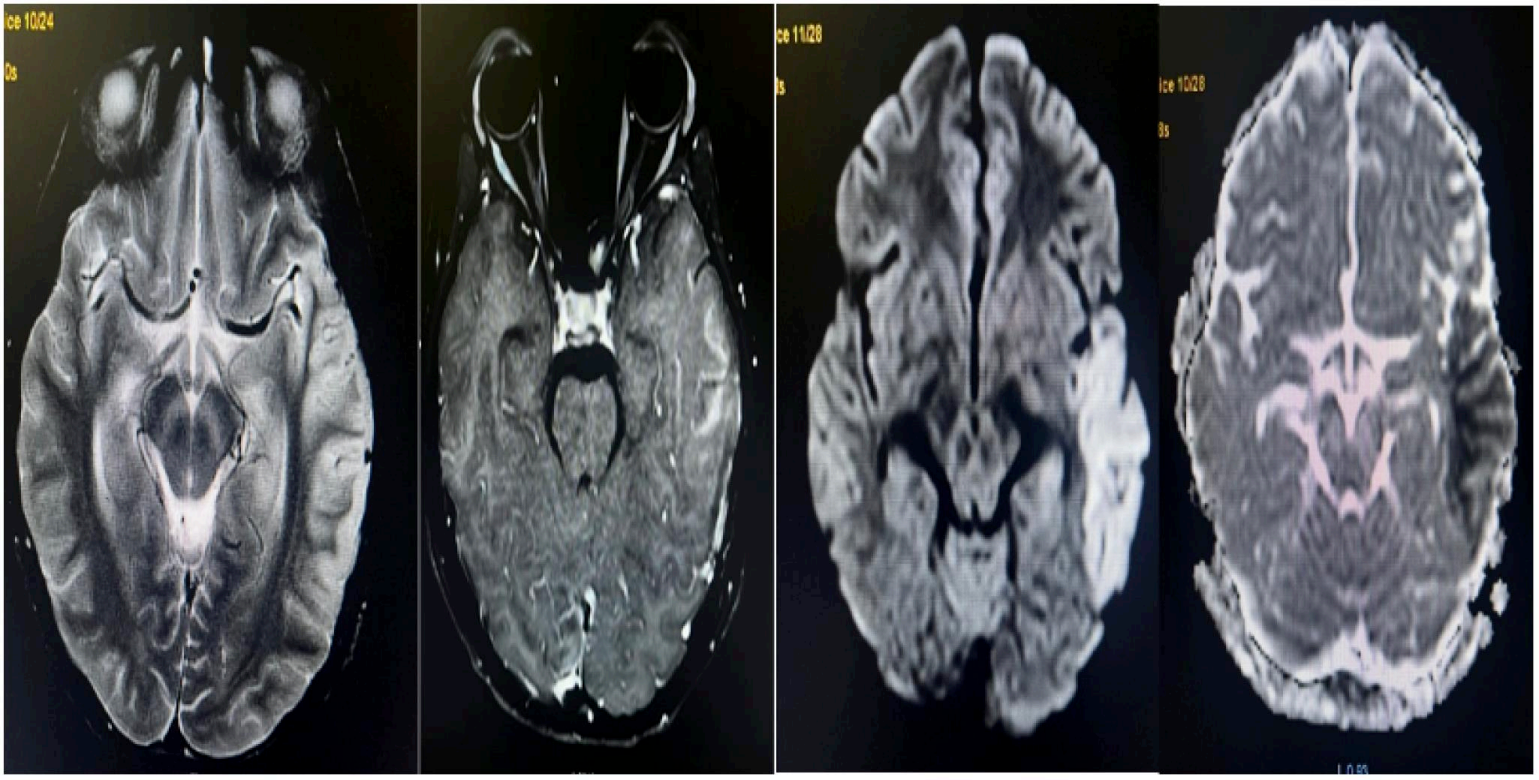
Axial T2 cortical hyperintensity in the left temporal region with gyriform enhancement in post-contrast T1 fat sat image. Corresponding areas show diffusion restriction with high signal on DWI with a low signal on ADC map.
